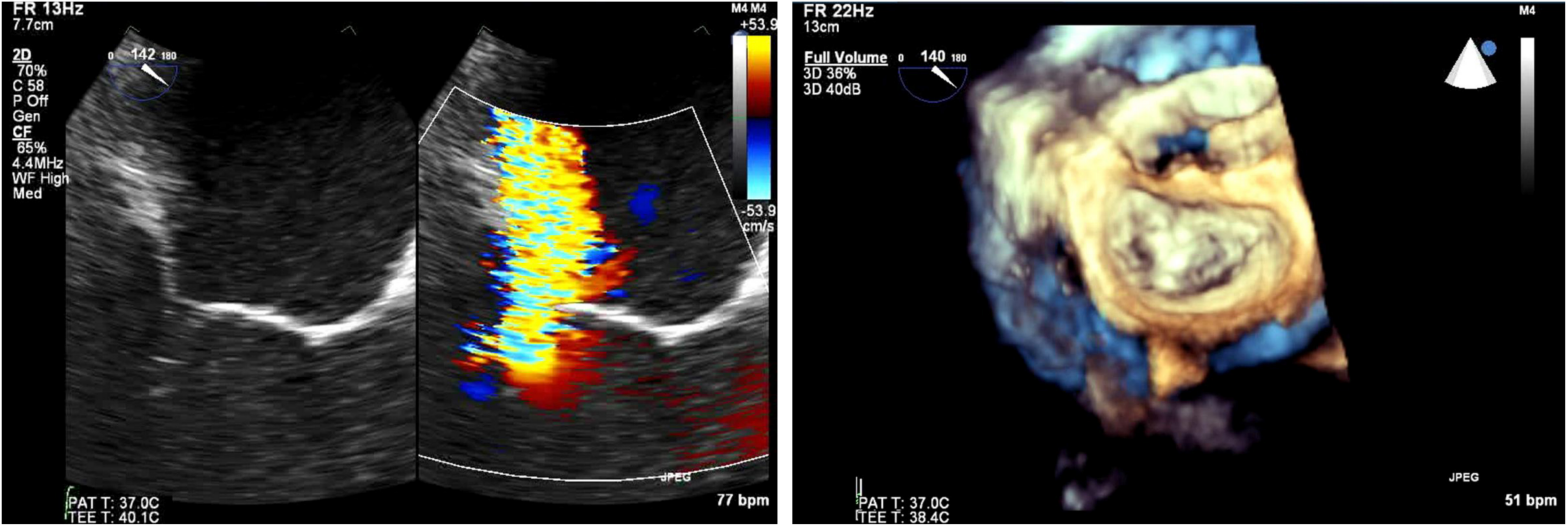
(Left) Tranesophageal echocardiography at 140 degrees for the evaluation of severe mitral regurgitation—(right) MitraClip positioning and 3D- transesophageal surgical view.
